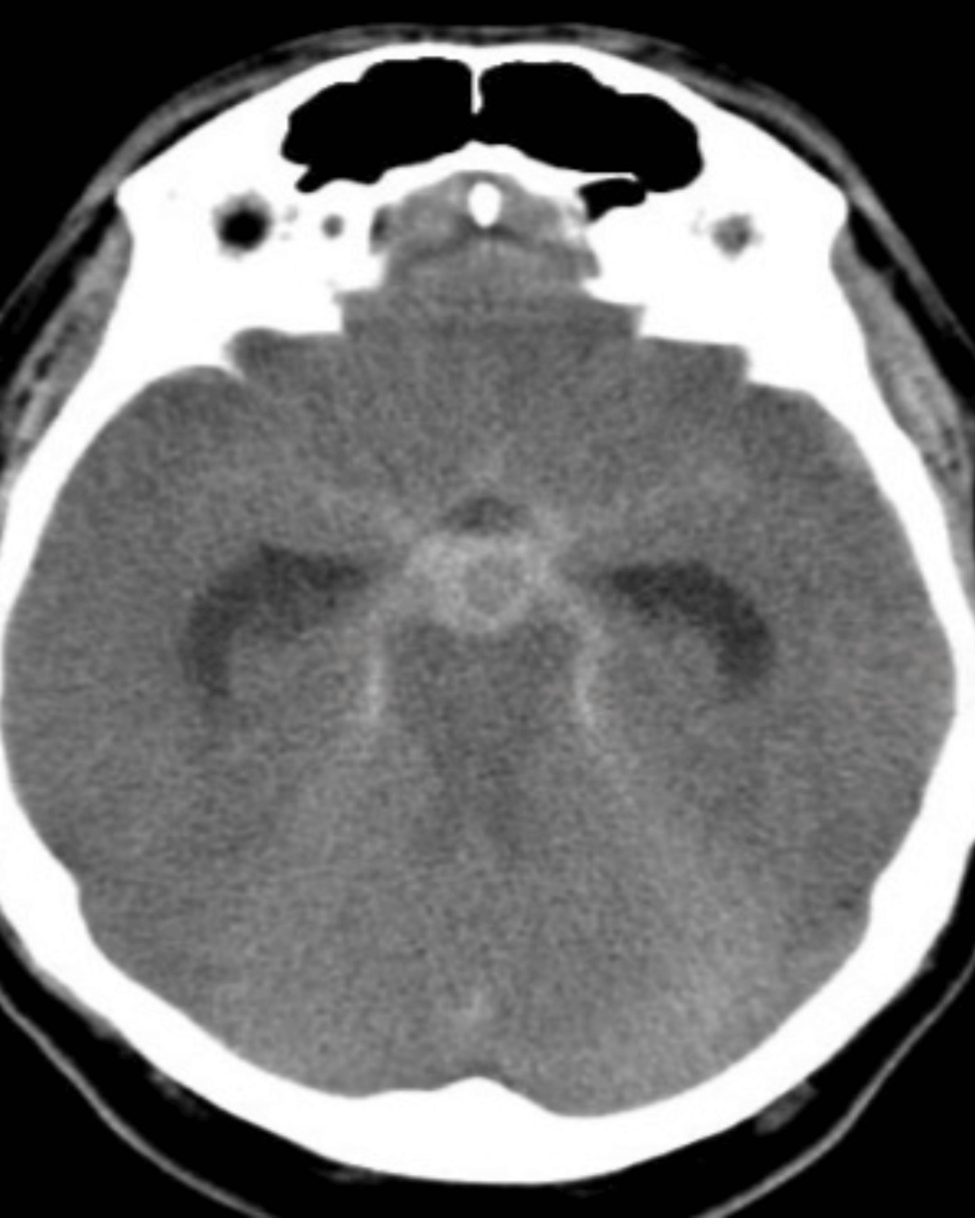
Non-contrast CT suggestive of subarachnoid hemorrhage in Sylvian and basal cisterns and ruptured anterior communicating artery aneurysm.
